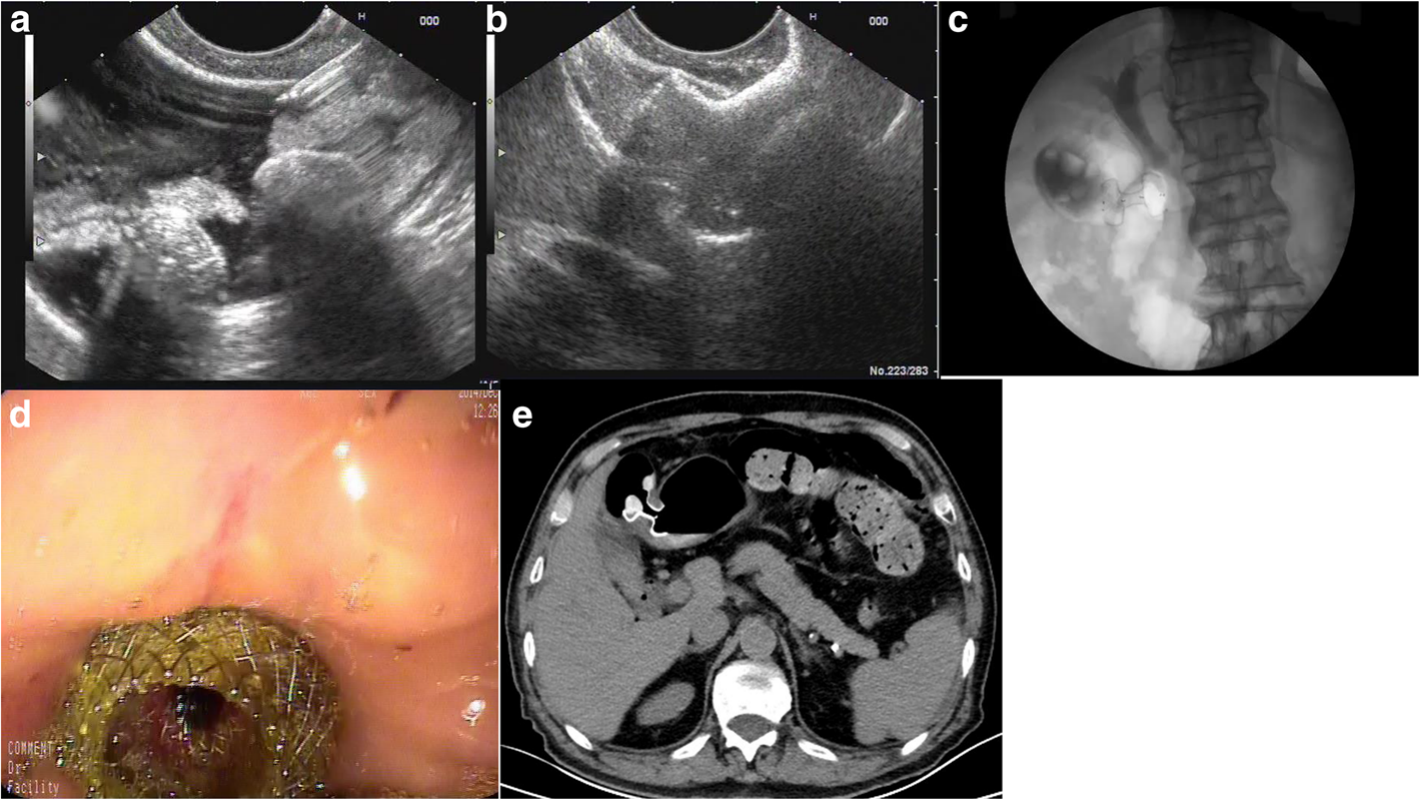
Cholecystogastrostomy. a EUS guided gallbladder puncture by echo-tip needle. b EUS guided stent releasing. c X-ray image of the released sent. d endoscopic view of the released stent. e CT exam flowing the cholecystogastrostomy
